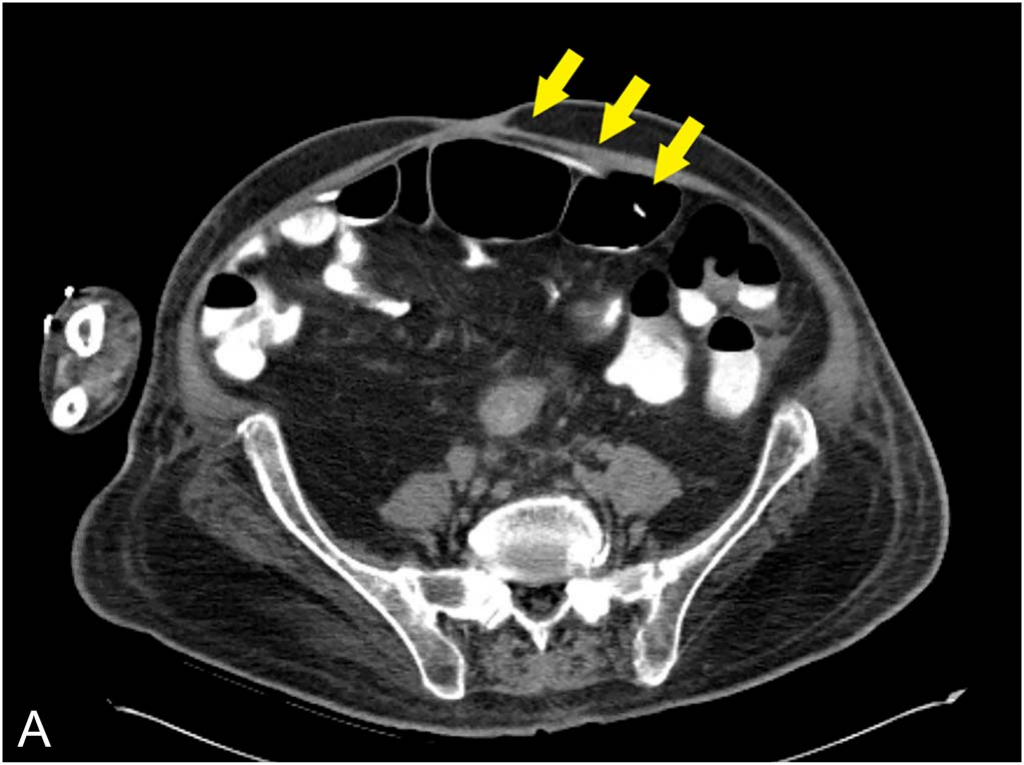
Abdominal computed tomography showing the syringoperitoneal shunt was terminating within the colon.
